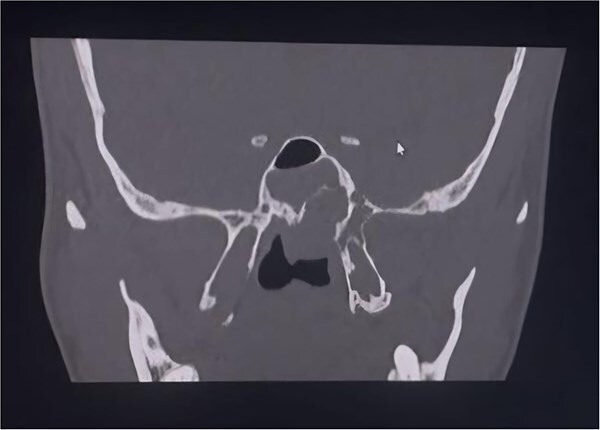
Coronal CT PNS (bone window) recurrent soft-tissue lesion centered at the left sphenopalatine/pterygopalatine region with posterior extension and adjacent bony remodeling erosion.
